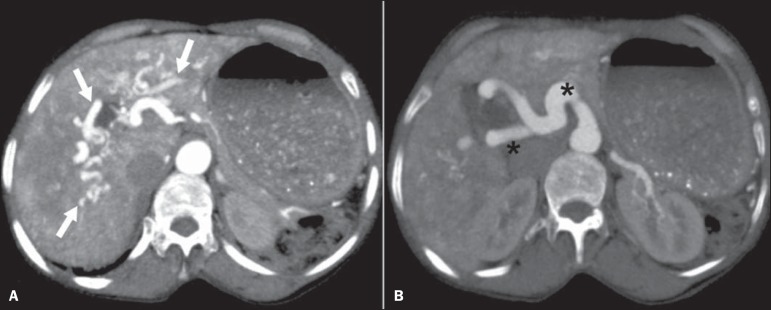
Rendu-Osler-Weber syndrome. Axial maximum intensity projection CT
